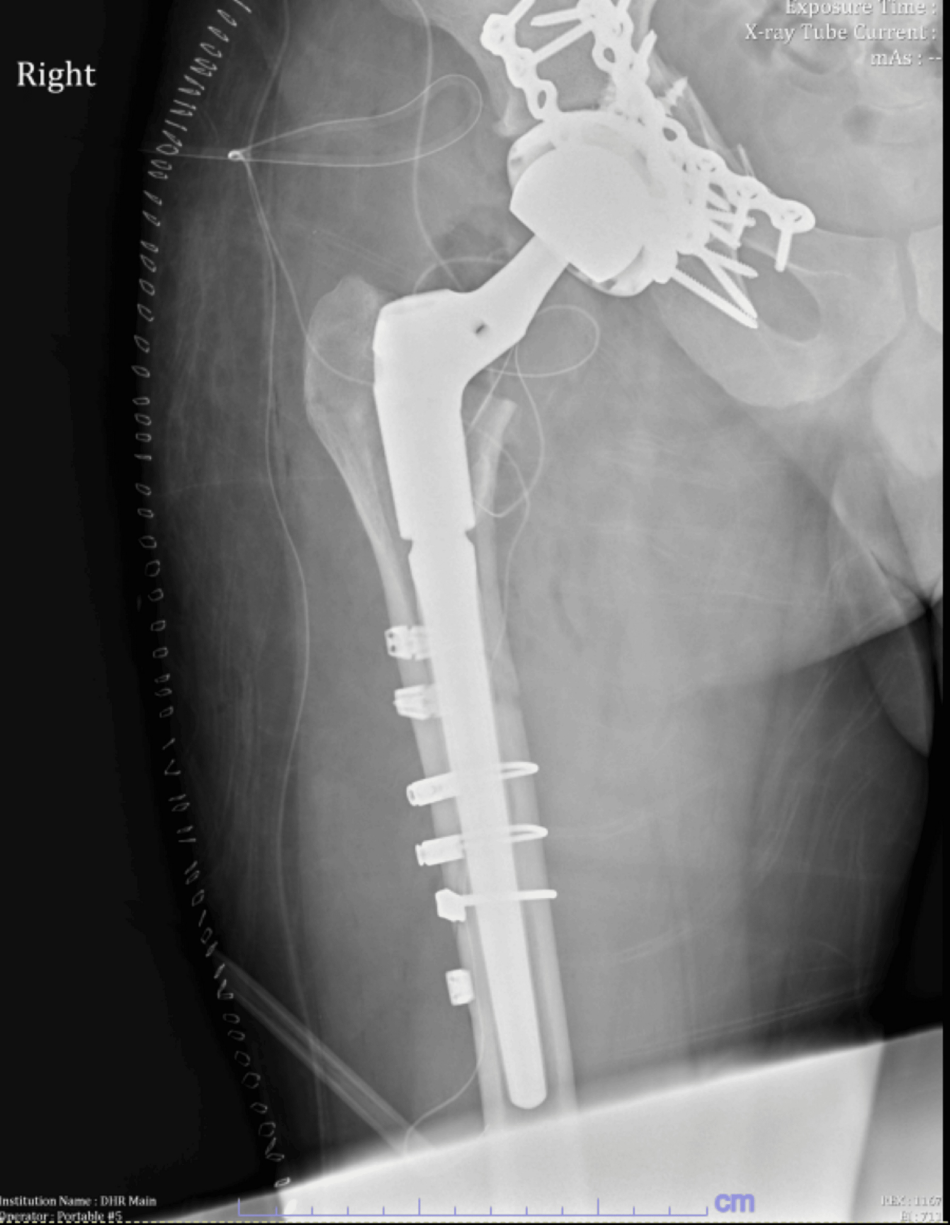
Postoperative AP X-ray of the right hip demonstrating long stem femoral prosthesis with a cerclage wire AP, anteroposterior
